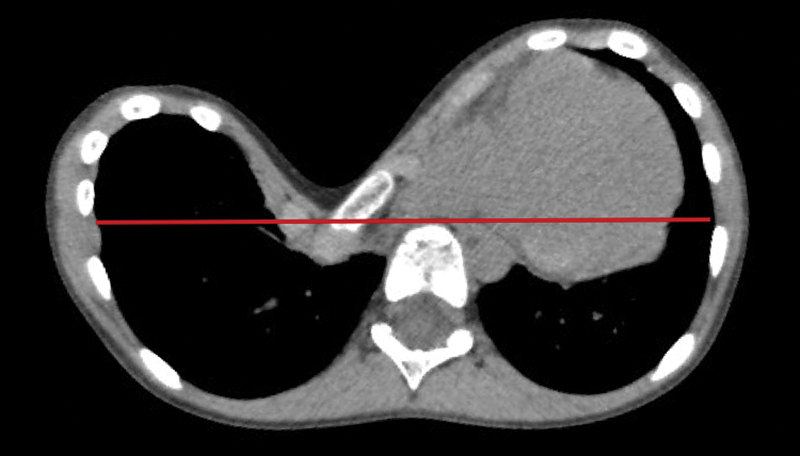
Patient's chest imaging prior to surgical correction. Chest computed tomography showing the sternum depression is below anterior vertebral column line (red line).
